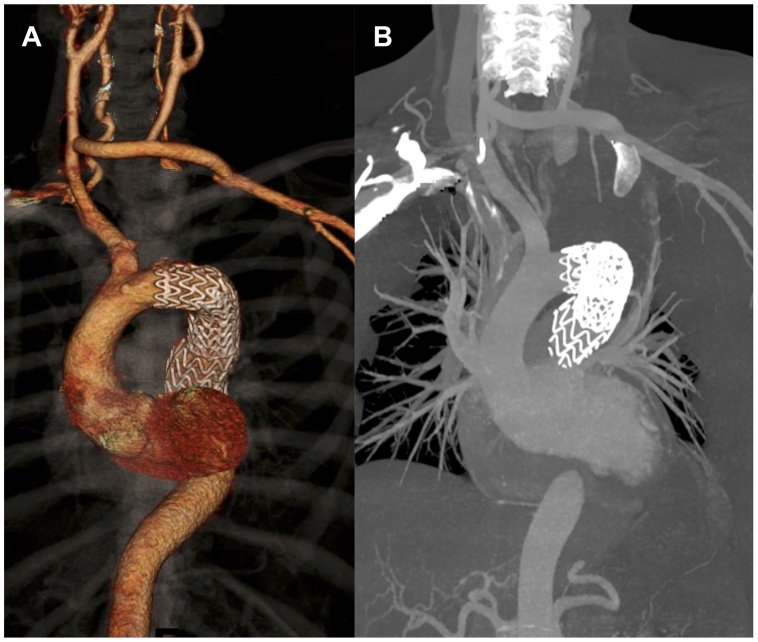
(A) Three-dimensional computed tomography angiography (CTA) reconstruction at the 5-year follow-up demonstrating durable exclusion of the pseudoaneurysm and configuration of the thoracic stent graft. (B) Coronal reconstruction showing patency of the carotid-carotid-subclavian bypass, preserved supra-aortic flow, and absence of endoleak or device-related complications.
